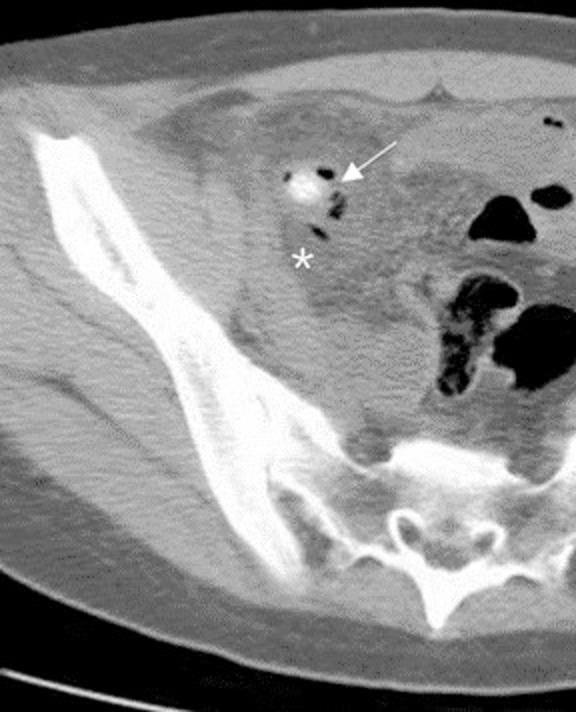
Periappendiceal air and extraluminal fluid. Axial CT images show an extraluminal air bubbles (arrow) mixed with fluid (asterisk) around the appendicolith
